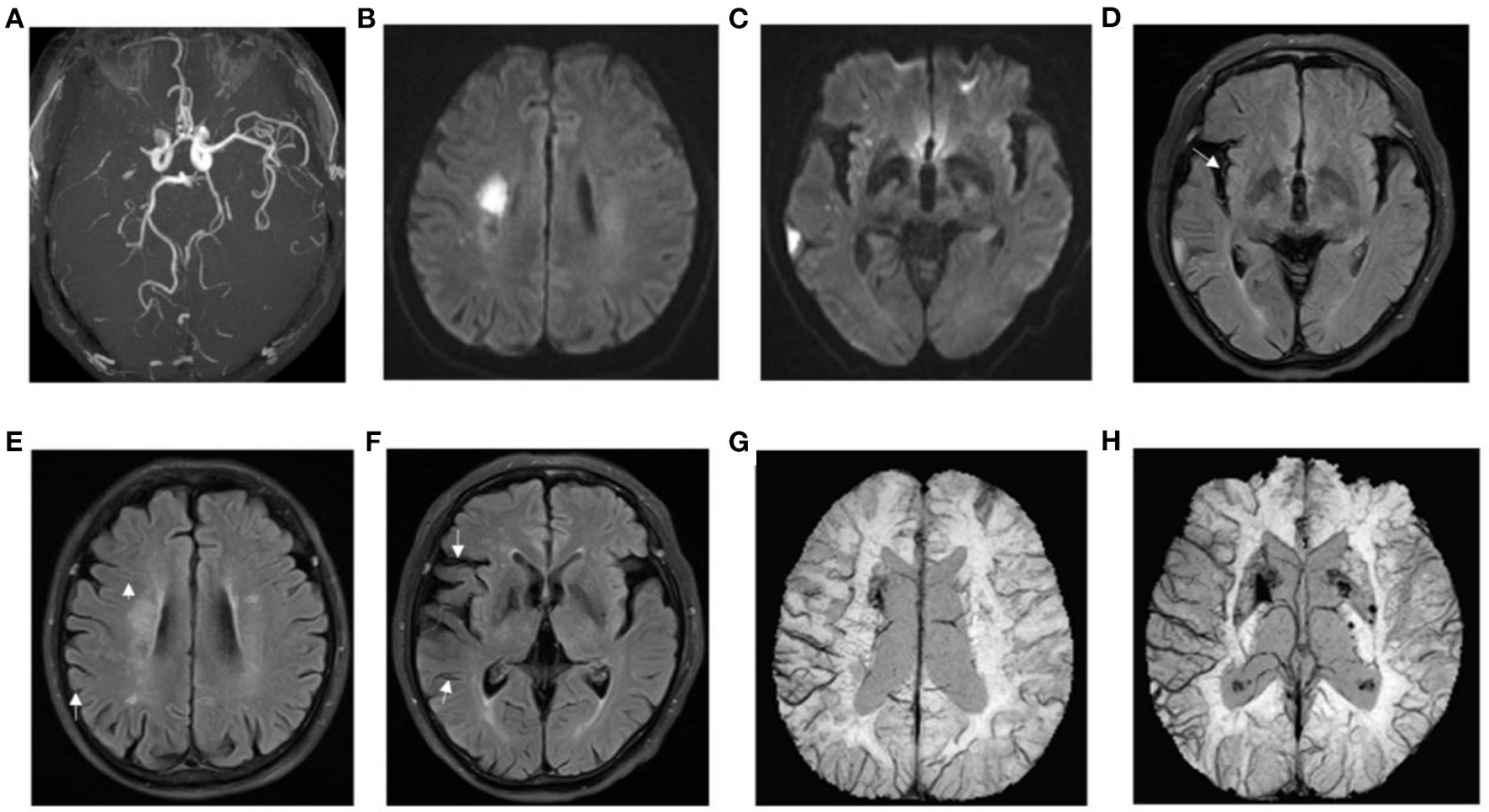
Presentation of a typical patient. A 62-year-old man with left limb weakness for 5 h. (A) right prominent laterality posterior (PLPCA) and anterior (PLACA) cerebral arteries; (B,C) diffusion-weighted imaging indicated acute cerebral infarction, and the DWI-ASPECTS was 7; (D–F) T2 fluid-attenuated inversion recovery (FLAIR) images showed hyperintense vessel sign (HVS), and a HVS score of 3; (G,H) susceptibility weighted imaging demonstrated prominent hypointensity vessel sign (PVS), and SWI-ASPECTS was 3. DWI-SWI mismatch score was 4. The patient had a favorable prognosis with a mRS of 1 at 90 days.
